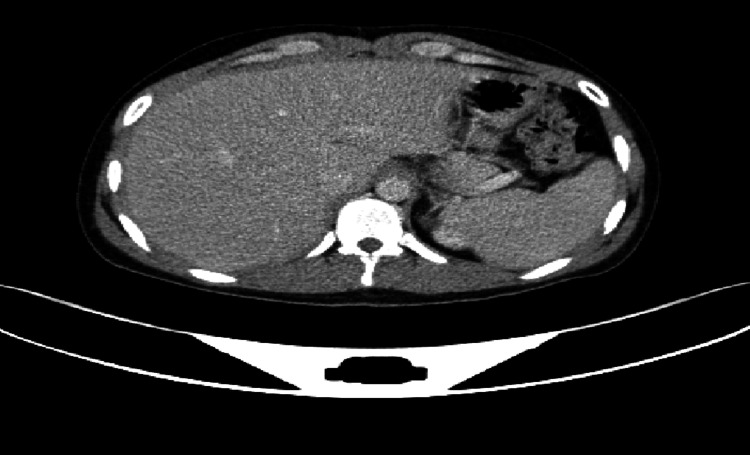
Contrast-enhanced CT of the abdomen demonstrating no significant abnormalities.
